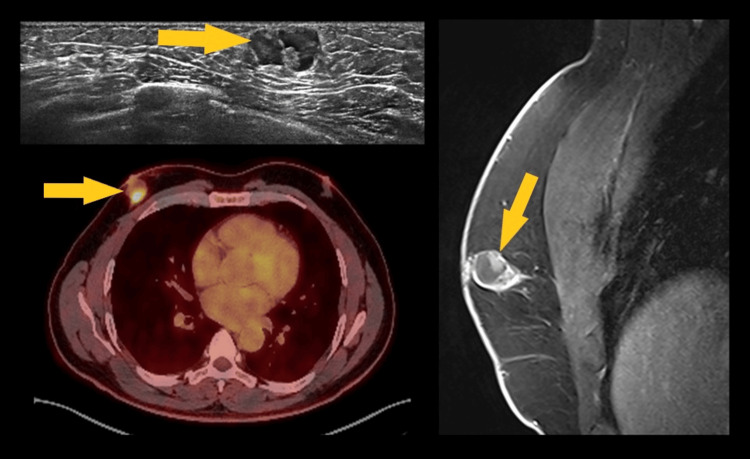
Transverse ABUS image (left top) shows the heterogeneous, well-bordered mass (papillary carcinoma). The mass uptakes the radiotracer on positron emission tomography (left bottom) and is observed as a complex cyst on MRI (right). The described lesion is highlighted with a yellow arrow.
